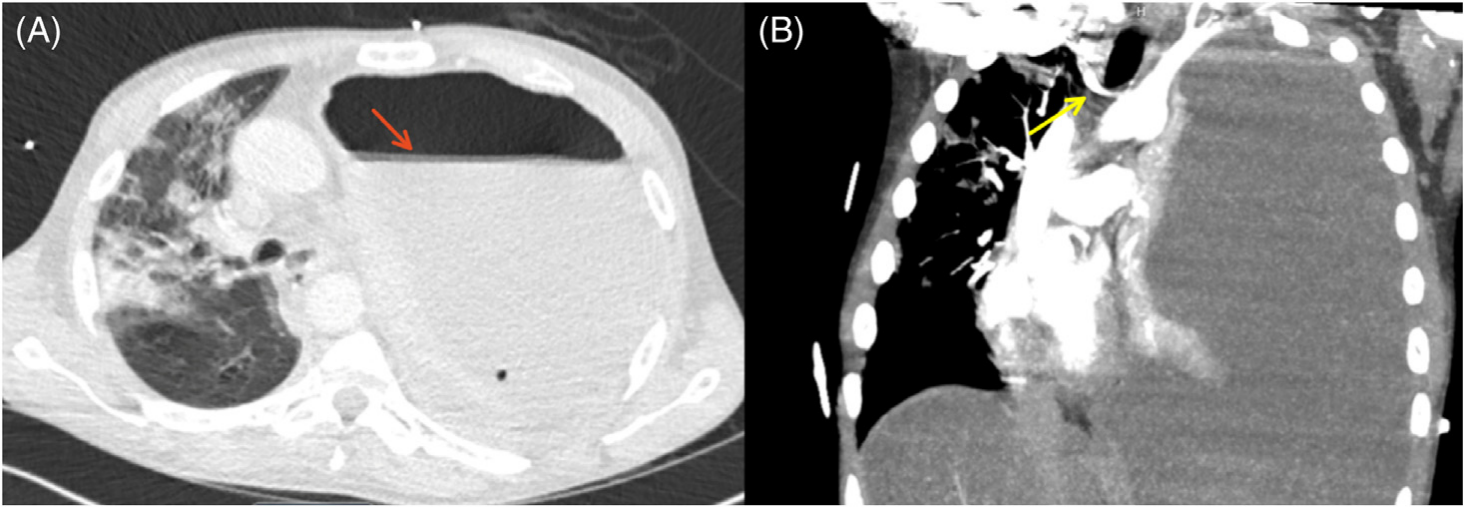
A. Axial view of initial computed tomography chest showing large left hydropneumothorax with complete left lung collapse (red arrow). B. Coronal view of significant rightward shift of the trachea and mediastinum (yellow arrow).
